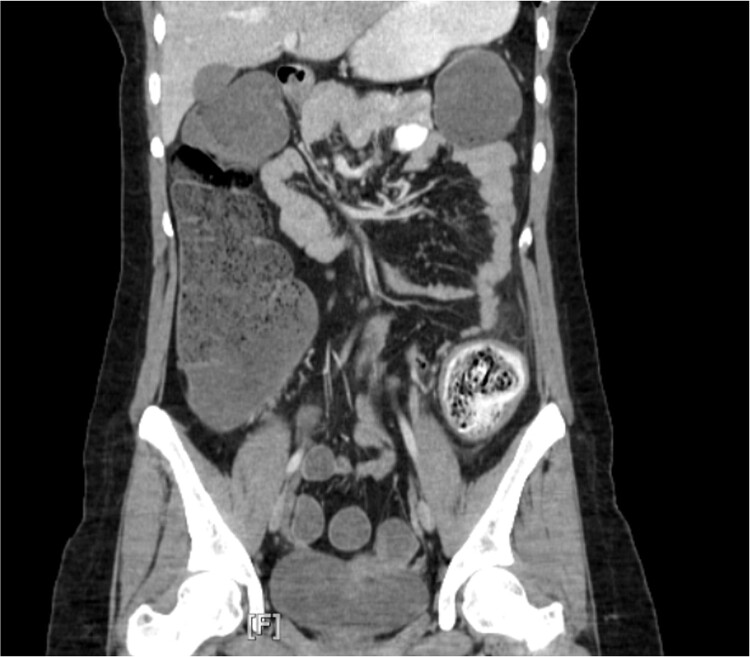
Computed tomography imaging revealed a mechanical bowel obstruction at the distal descending colon secondary to impacted fecal matter with noted colonic wall thickening and edema.
